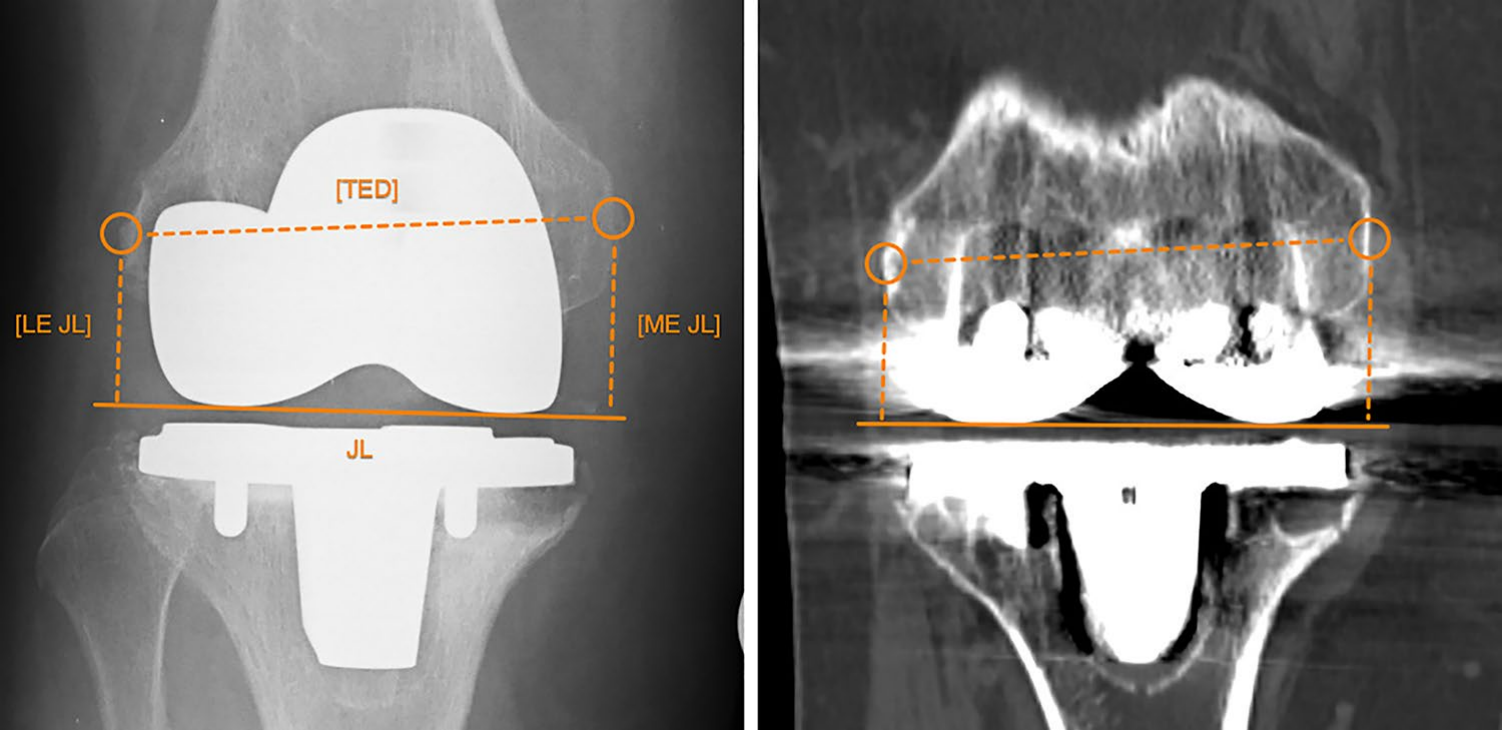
The epicondylar ratio. Measurement of the LER and MER in X-ray on the left and CT on the right. *TED* transepicondylar distance, *JL* joint line, *LE JL* distance between the lateral epicondyle and the joint line, *ME JL* distance between the medial epicondyle and the joint line
